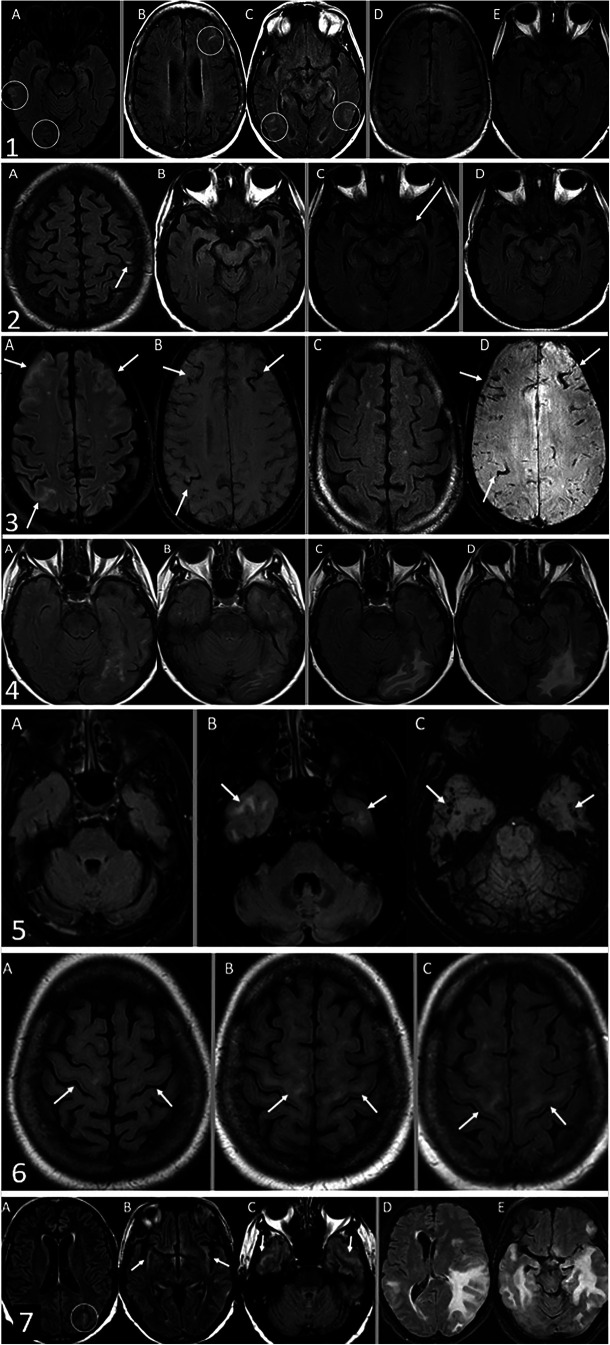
Case 1. ARIA-E with interval resolution. The baseline MRI did not demonstrate any acute findings, only chronic atrophy and microvascular angiopathy (not shown). The safety MRI by FLAIR sequence (A) 42 days after baseline MRI revealed interval development of sulcal FLAIR hyperintensities compatible with sulcal effusions in the right temporal and occipital lobes. Thirty-two days later, the first follow-up MRI (B and C) revealed new sulcal effusions in the left frontal, left posterior temporal, and right temporal-occipital lobes. Subsequent MRI after another 58 days (D and E) demonstrated complete interval resolution of these sulcal effusions. Case 2. ARIA-E. The safety MRI by FLAIR sequence (A) revealed interval development of a tiny sulcal effusion in the left frontal lobe, compatible with ARIA-E. Of note, the medial temporal lobes were normal (B) 26 days later, the first follow-up MRI (C) revealed a new subtle FLAIR hyperintensity in the medial left temporal lobe. Subsequent MRI after another 31 days (D) demonstrated interval resolution of FLAIR hyperintensity in the medial left temporal lobe. Case 3. ARIA-E and H. The safety MRI (A: FLAIR sequence and B: SWI sequence) revealed interval development of sulcal effusions in the bilateral frontal and right parietal lobes. Trace subarachnoid hemorrhages are also evident at the same locations (B) Follow-up MRI after 70 days (C: FLAIR sequence and D: SWI sequence) demonstrated interval resolution of sulcal effusions but persistent sulcal hemosiderin deposition (D) Case 4. ARIA-E by FLAIR sequence. The safety MRI (A and B) revealed interval development of vasogenic edema in the left occipital lobe. Follow-up MRI after 30 days(C and D) demonstrated worsening edema. Case 5. ARIA-E and H. A baseline FLAIR sequence (A: FLAIR sequence) is unremarkable. The safety MRI revealed interval development of vasogenic edema (B: FLAIR sequence) and microhemorrhages (C: SWI sequence) in the bilateral anterior temporal lobes. Case 6. ARIA-E with FLAIR hyperintensity involving the subcortical corticospinal tracts as a manifestation of ARIA. Baseline MRI (A) demonstrated normal signal intensity of subcortical corticospinal tracts. The first follow-up MRI after 98 days revealed interval development of symmetrical FLAIR hyperintensities in the subcortical corticospinal tracts (B), which remain unchanged on subsequent MRI obtained three months later (C) Case 7. Severe ARIA-E by FLAIR sequence. The safety MRI (A-C) revealed trace sulcal effusion in the left occipital lobe (A) and minimal FLAIR hyperintensities in the bilateral insular cortices and anterior temporal lobes (B and C). Seven months later, the patient presented with behavior and cognition changes as well as nighttime confusion with hallucinations. A follow-up MRI performed at that time (D and E) demonstrated marked worsening of multifocal edema of the temporal lobes with new foci in the left frontal lobe, associated mass effect, effacement of left lateral ventricle, new left-to-right midline shift, and left uncal herniation.
